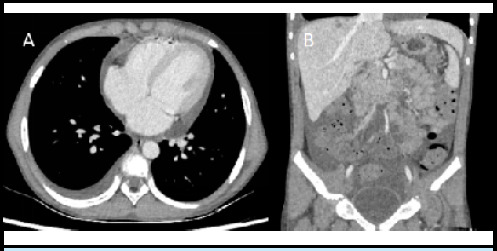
Contrast enhanced CT axial section (A) showing mild right pleural effusion and pericardial effusion. Coronal section (B) showing ascites in abdomen.
